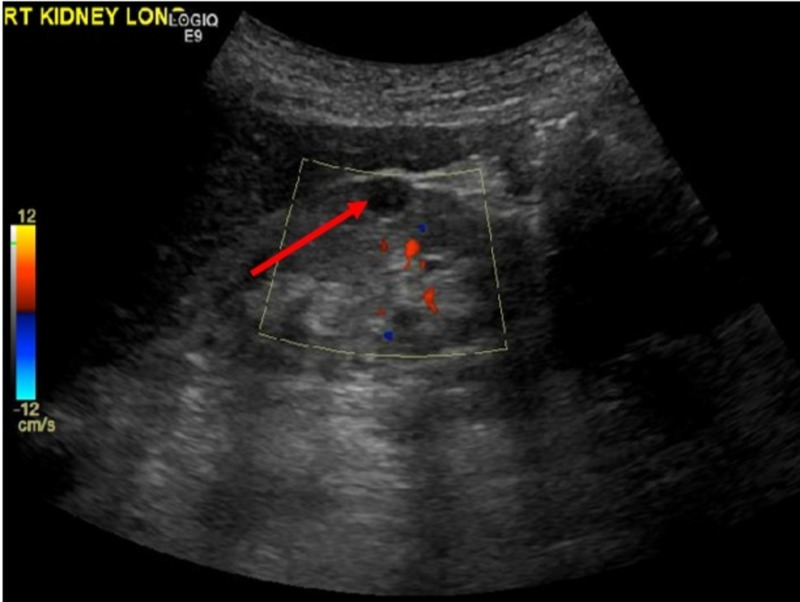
Ultrasound of right kidney. Arrow indicates 0.8 x 0.9 x 0.9 cm cystic lesion in mid-pole region.
